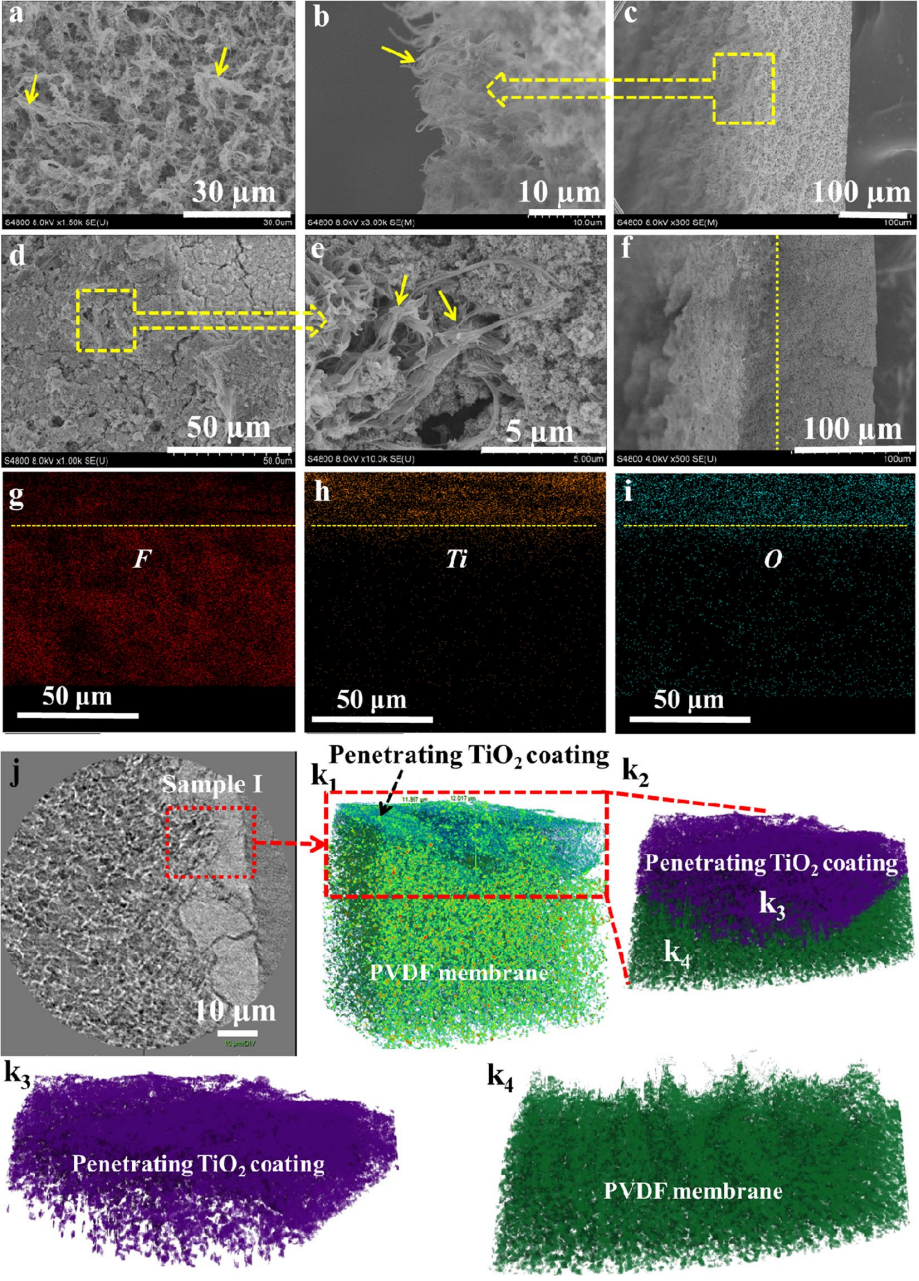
Structure and chemical components of the pristine PVDF membrane (a–c) and nano-TiO_2_ manipulated membrane (d–f). (a,c) SEM images of the pristine PVDF membrane surface and cross-section. (b) Enlarged SEM image of the pristine PVDF membrane cross-section. (d,f) SEM images of the nano-TiO_2_ manipulated membrane surface and cross-section. (e) Enlarged SEM image of the nano-TiO_2_ manipulated membrane surface. (g,h and f) EDX mapping of F, Ti and O elements distribution for nano-TiO_2_ manipulated membrane cross-section. (j) Overview of nano-TiO_2_ manipulated membrane for nano-CT. (k_1_–k_4_) 3D reconstructed and stripped microstructure of Sample I.
